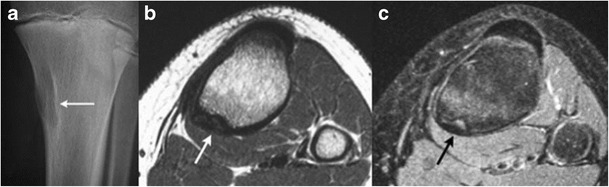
Fibrous cortical defect. A 13-year-old boy with a fibrous cortical defect in the proximal right postero-medial tibia. An antero-posterior radiograph of right tibia a shows an intra-cortical lucency with a thin rim of sclerosis (arrow). Axial T1 b and T2 fat-suppressed c images show a hypointense intracortical lesion with no medullary involvement. A hyperintense rim is seen on the T2-weighted image (*black arrow*)- JuxtacorticalJuxtacortical/Periosteal chondromaThese are benign protuberant hyaline cartilaginous tumours, which arise from the periosteum and are superficial to the bone cortex. Juxtacortical chondromas are rare lesions, accounting for < 1 % of overall bone lesions. More common in young adults, the exact incidence in childhood is unknown, although they have been reported in children [12]. The metaphyseal ends of long bones and small bones of the hands and feet are commonly involved.Radiologic features include a scalloped cortical surface with or without sclerosis, a thin periosteal shell and chondroid mineralization of the matrix [8]. On MR, typical signal characteristics of chondral tissue are noted, with an intermediate signal on T1WI and a heterogeneously hyperintense signal on T2WI [8]. Low-signal borders on T2WI and gradient recall echo (T2*WI) are often present, representing thin sclerotic reaction. Patchy matrix mineralization can contribute to interspersed areas of low signal on T2WI. Reactive perilesional or intramedullary oedema is not typical. Thin peripheral and septal contrast enhancement may be seen (Fig. 6) [8].
